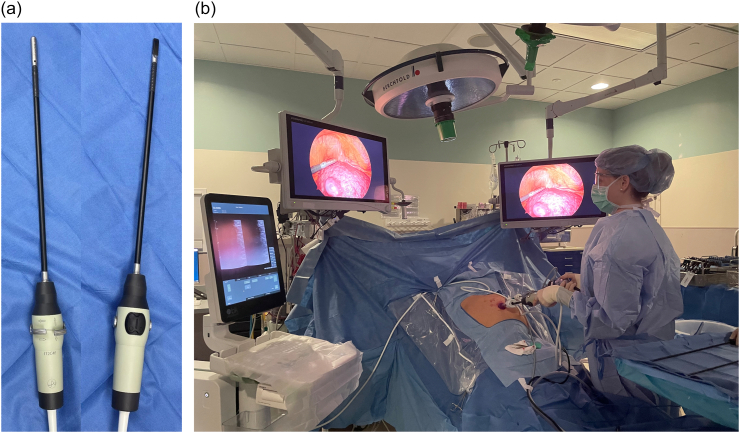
a) Flexible linear probe for laparoscopic intraoperative ultrasound, b) Typical operating room setup for performing laparoscopic liver ultrasound.
